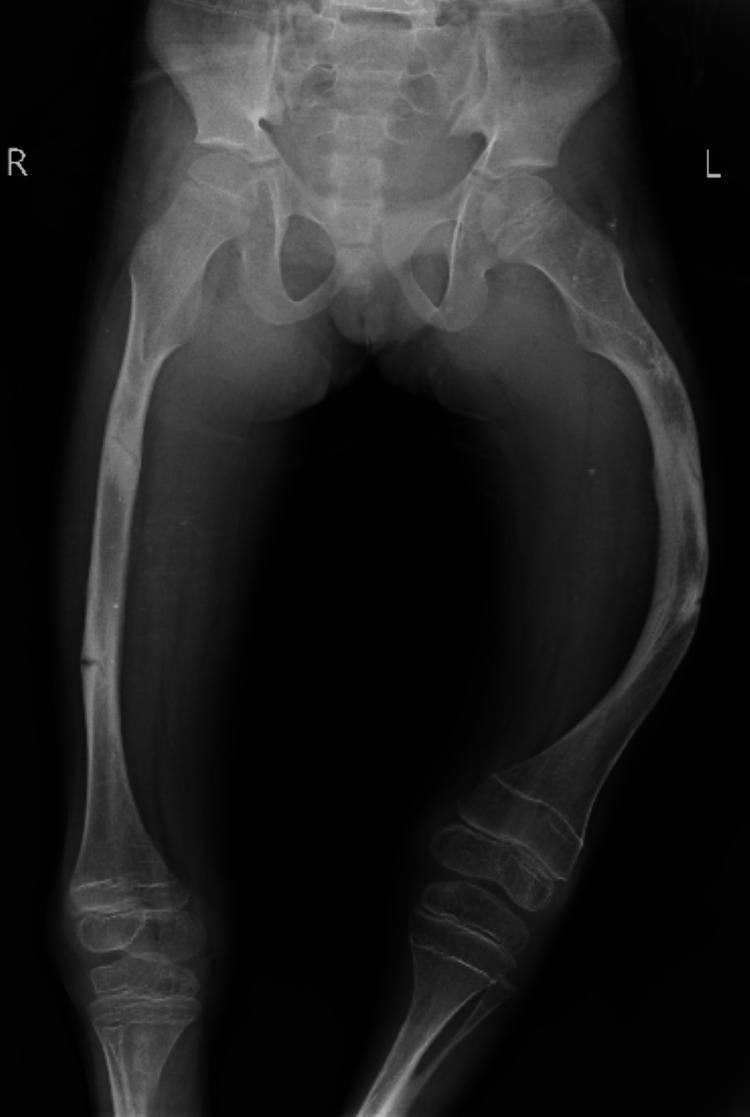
X-ray of the right femur.
